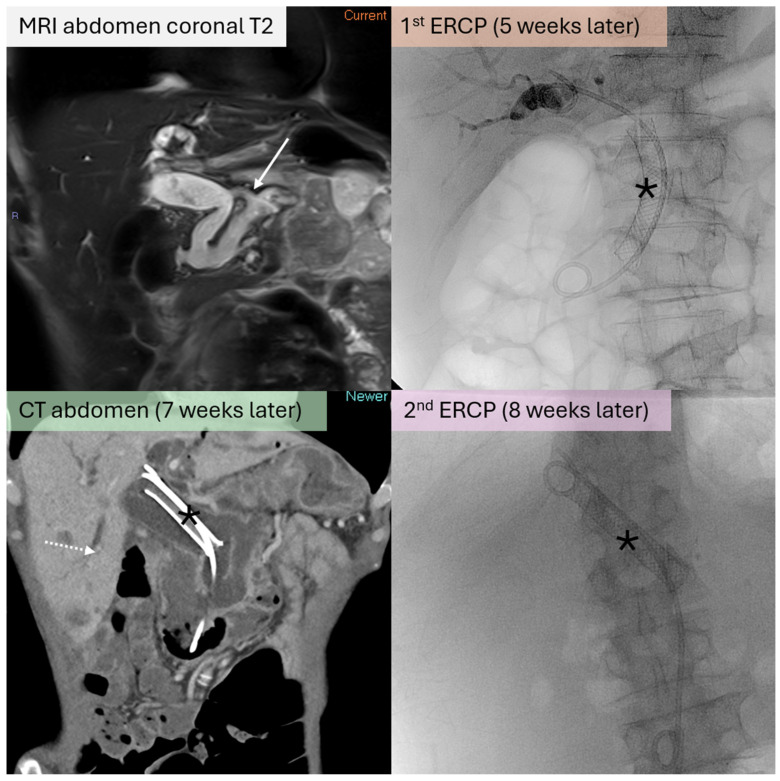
(Top left) Surveillance MRCP study (coronal T2 sequence) showing fistulous communication between dilated MPD (white arrow) and upper CBD. (Top right) Plastic double pigtail and fully covered metallic (black asterisk) biliary stents placed endoscopically during endoscopic retrograde cholangiopancreatography (ERCP). (Bottom left) Repeat CT performed for obstructive jaundice showed proximal migration of the covered metallic stent (black asterisk) with resultant intrahepatic biliary dilatation (dashed white arrow). (Bottom right) Repeat ERCP confirmed proximal migration of the metallic stent (black asterisk). Stent was removed and exchanged (not pictured).
